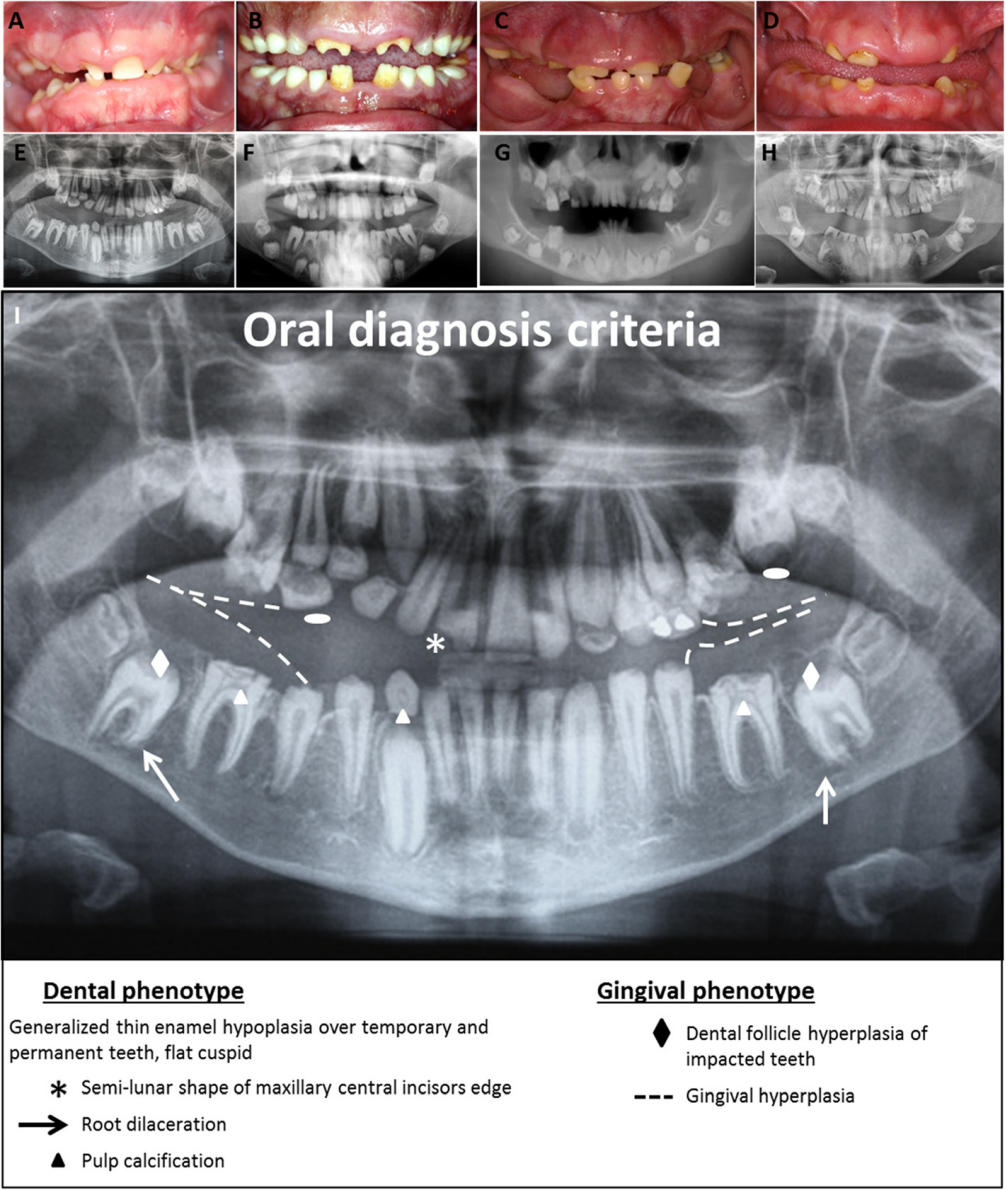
Pathognomonic oral phenotypes in patients with Enamel Renal Syndrome. (A, B, C, D, E, F, G, H) Oral views and corresponding orthopantomogram of 4 unrelated ERS patients, all with recessive mutations in *FAM20A*. Oral view showing hypoplastic AI, delayed tooth eruption, widely spaced teeth, flat cusps of posterior teeth, upper permanent central incisor crown resorption and gingival hyperplasia. Orthopantomograms show generalized absence of enamel, altered tooth eruption pathway, flat cusps of posterior teeth (white elipse), intra-pulpal calcification (white triangle), hyperplastic dental follicles (white diamond), and gingival hyperplasia (dashed line). Semi-lunar shape of the permanent right upper central incisor, crown resorption and root dilacerations (white arrow) are also present. Note the consistent oral features in all patients. (I) Summary picture of pathognomonic oral diagnosis criteria listed in orthopantomogram.
